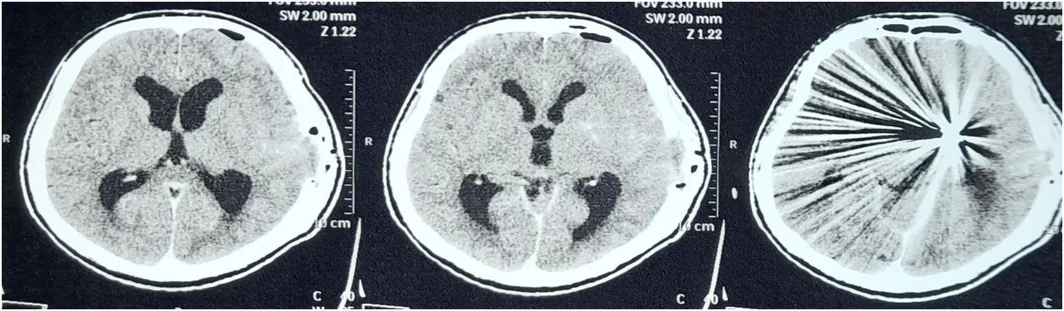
Multislice head CT axial sections, after endovascular exclusion of a fusiform aneurysm of the left middle cerebral artery using detachable microcoils. The high‐attenuation coil mass is seen within the treated aneurysm with associated beam‐hardening artifact. There is no new intraparenchymal hemorrhage and no established territorial infarction on these sections.
